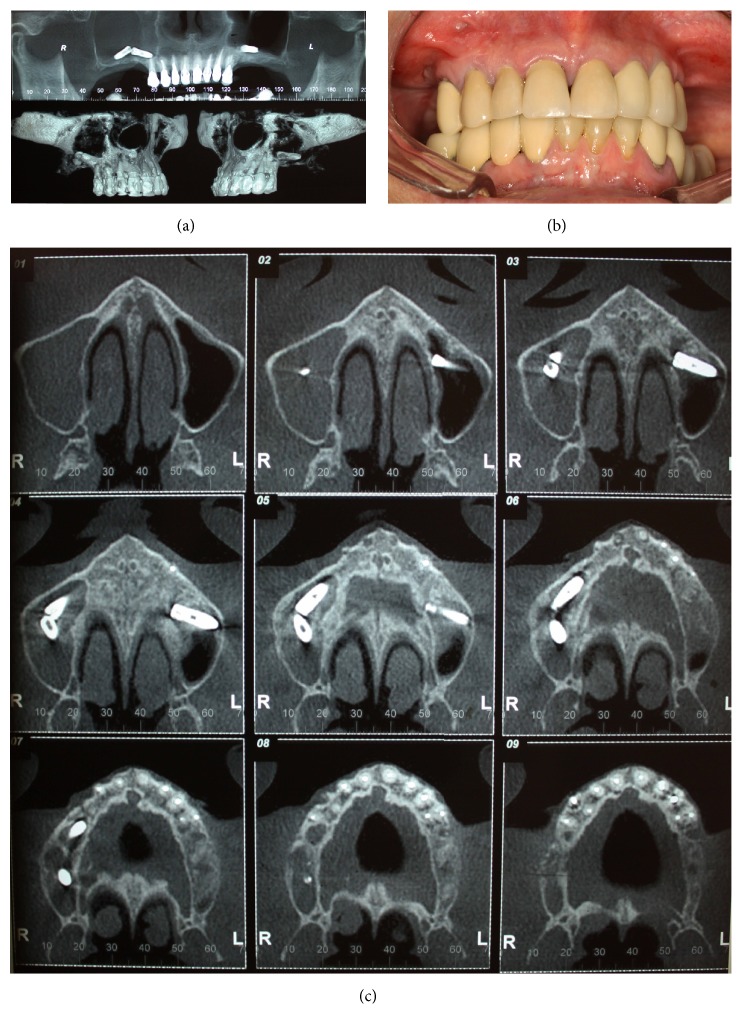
(a) A panoramic and a tridimensional reconstruction CT scan showing the implants displaced into the maxillary sinus cavity with a significant opacification of the right maxillary sinus; however, the left sinus does not present the opacification. (b) Photography showing the frontal clinical view. (c) A CT scan in axial sections showing implant displacement into the maxillary sinus cavity with a significant opacification of the right maxillary sinus. The left sinus does not present the opacification.
